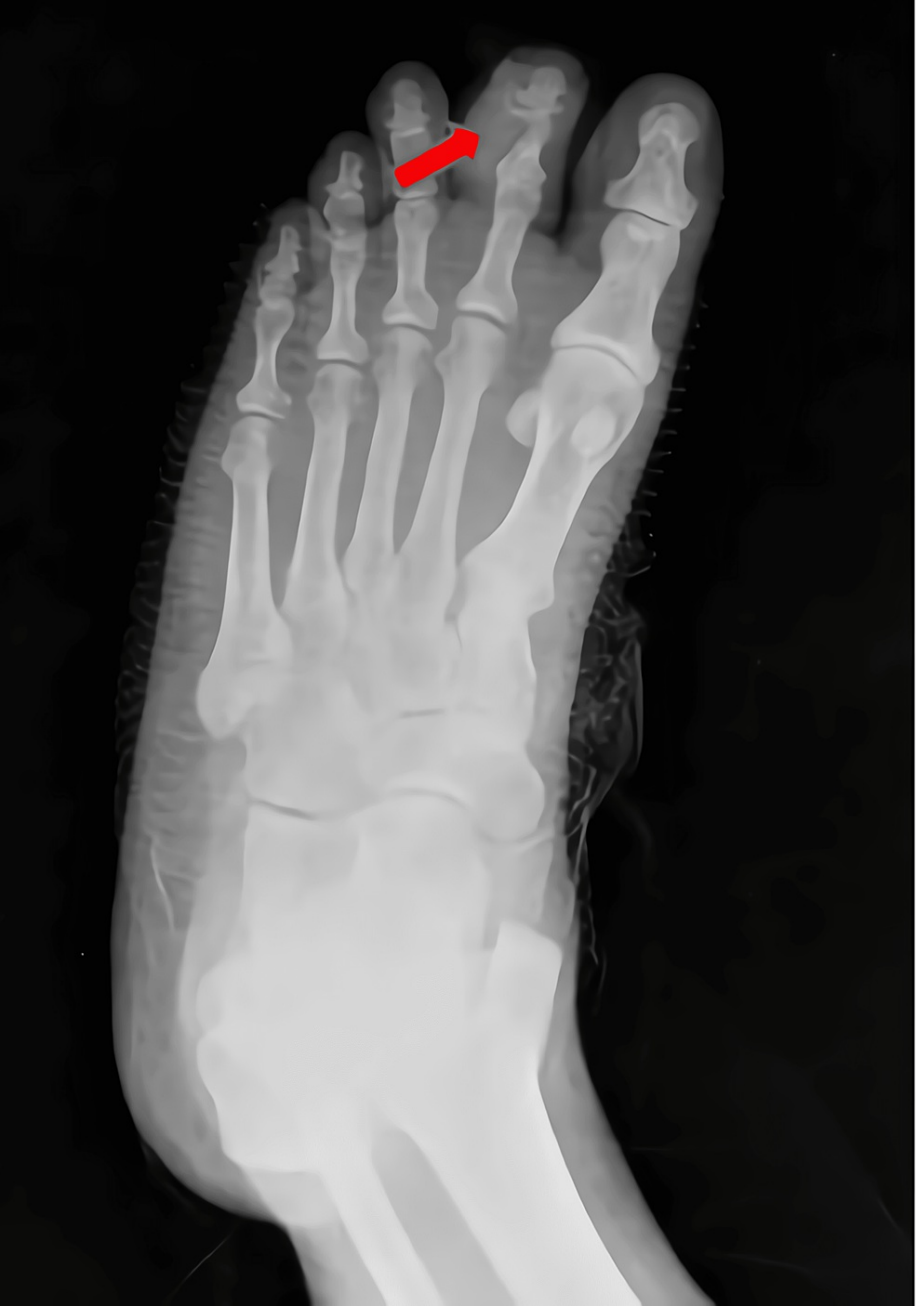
Postoperative X-ray image.
